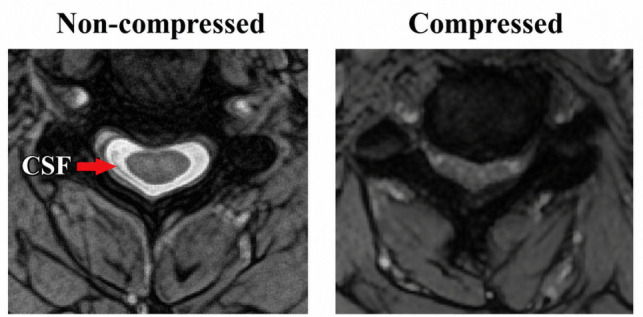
Axial SC T2* Weighted MRI in non-compressed (left) and compressed (right) conditions, showing reduced boundary contrast under compression.
